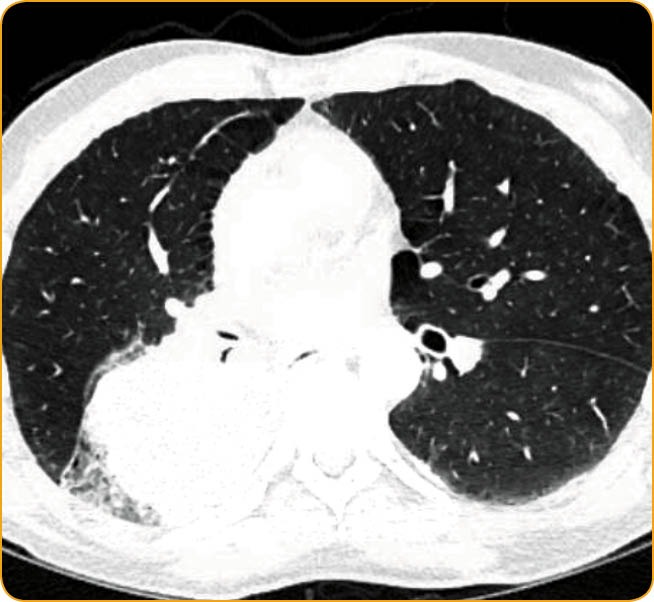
CT lung window shows the lung parenchyma
